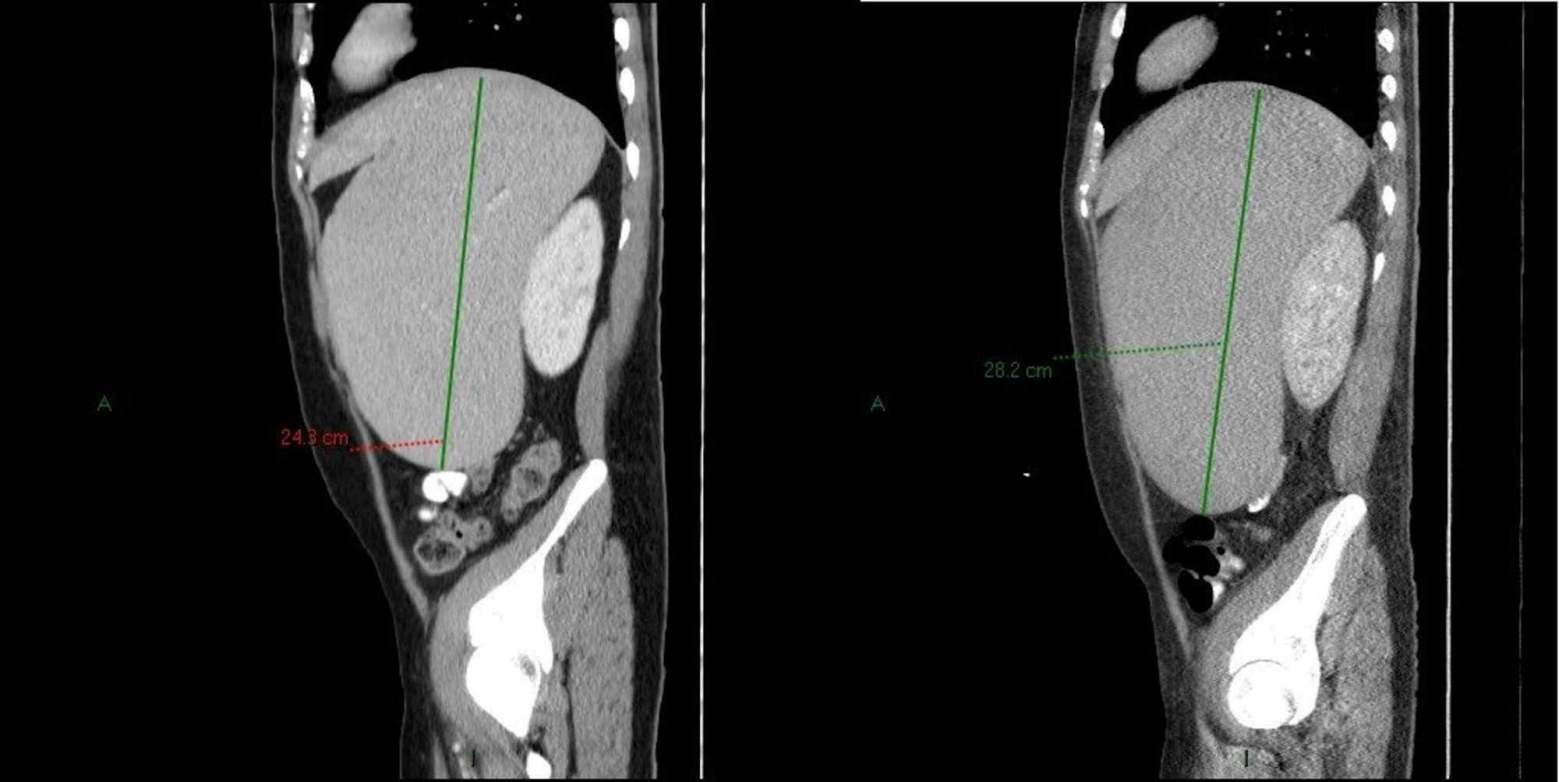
Sagittal section of CT scan showing rapidly progressing splenomegaly with a comparison of two studies done approximately two weeks apart. The spleen can be seen to have enlarged from 24.3 cm (left) to 28.2 cm (right).
